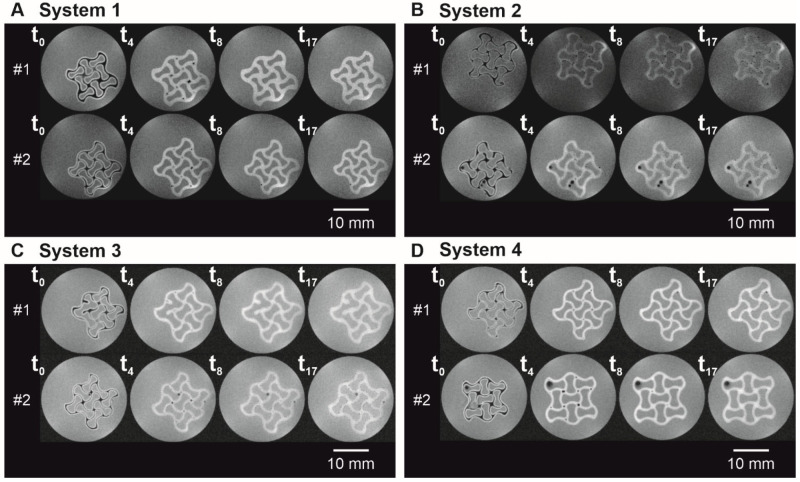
Auxetic structures in different liquid phases in MRI grayscale images with a field of view of 25 × 25 mm² at distinct time points. The liquid phase was (A) System 1, (B) System 2, (C) System 3, and (D) System 4 (*n* = 2).
